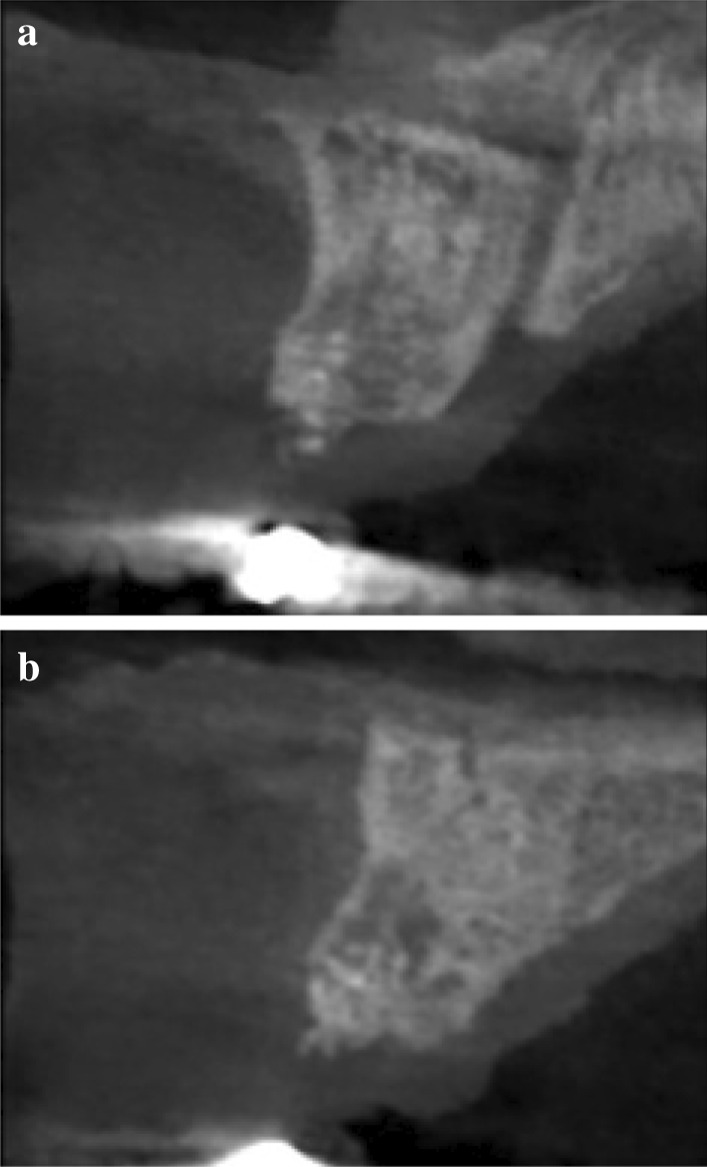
6-month postoperative CBCT scan before re-entry, (a) upper right first incisor, (b) upper left first incisor
